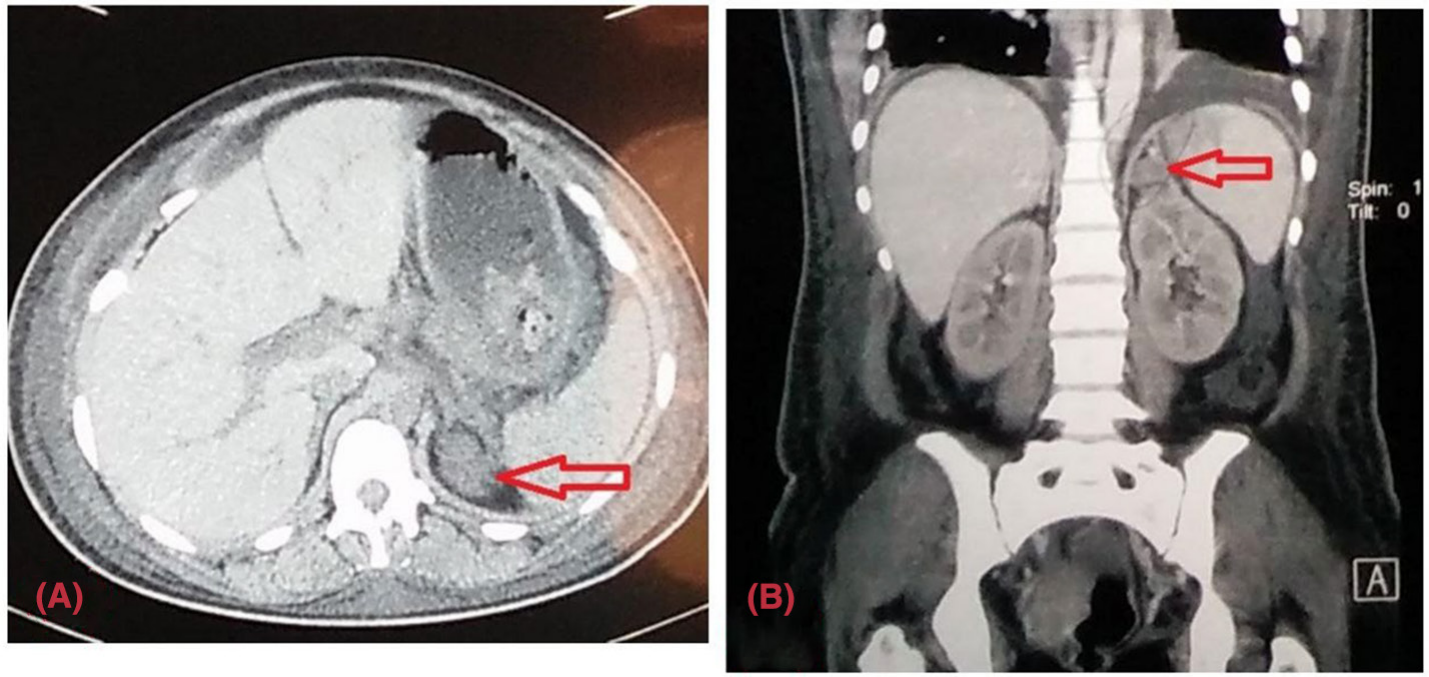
(A) CT coronal view showing supra renal mass (B) CT axial view showing adrenal mass
